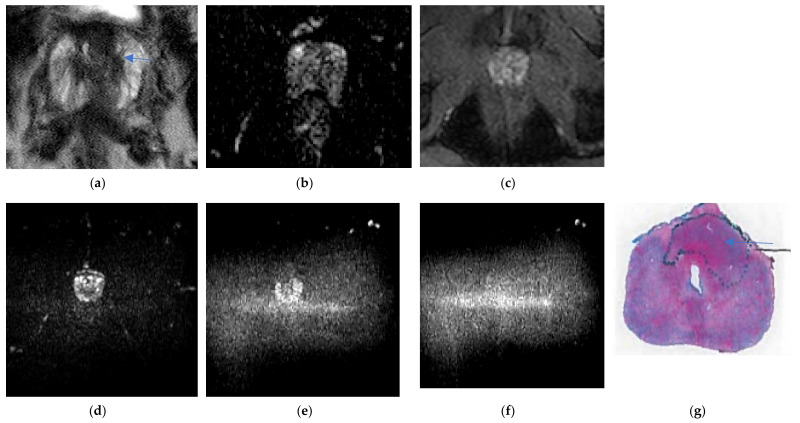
NERC unable to clearly identify extra-prostatic extension: a 64-year-old man, PSA = 7.2 ng/mL, Gleason 4 + 3 anterior mid-gland transition zone with extra-prostatic extension demonstrated as T2 hypo-intensity on Philips Achieva 3 T NERC T2WI (a), and indicated on whole mount pathology (g), undetected on NERC ADC (b), NERC DCE (c), NERC DWI b = 50 s/mm^2^ (d), NERC DWI b = 900 s/mm^2^ (e), NERC DWI b = 1500 s/mm^2^ (f). Images courtesy of University of Chicago.
